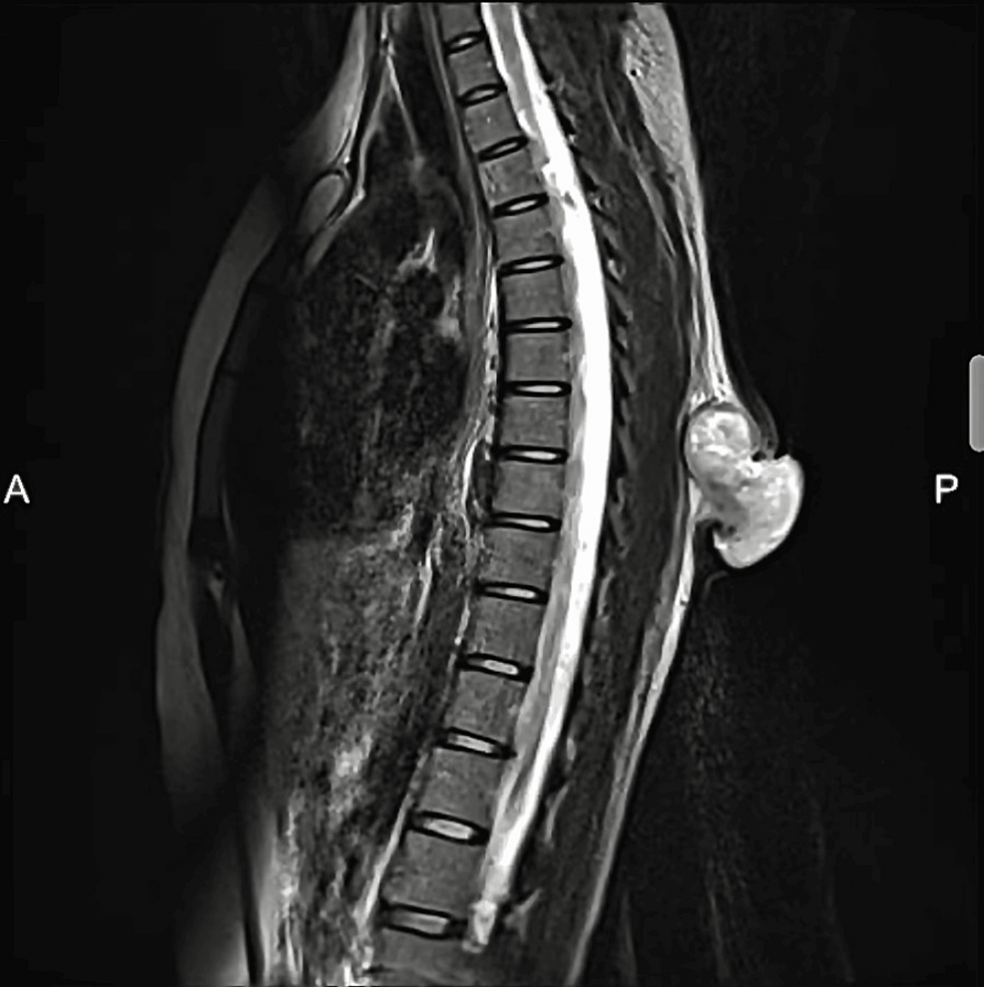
MRI spine sagittal T2 view showing a heterogenous exophytic mass over the subcutaneous soft tissue plans at posterior midline mid thoracic with contrast enhancement suspicious for infiltration
